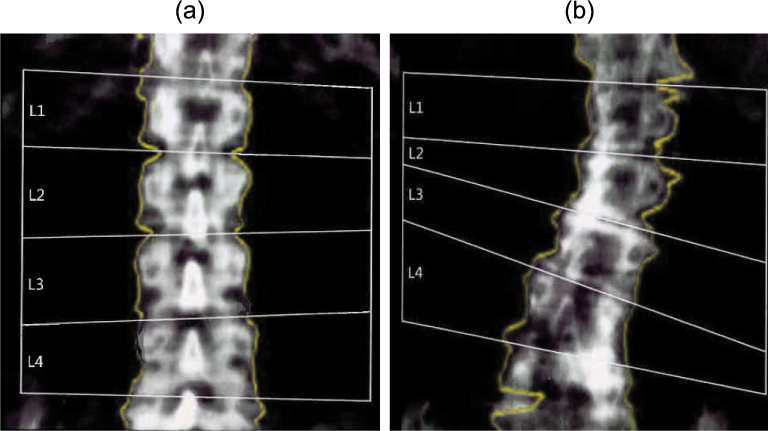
Dual-energy X-ray absorptiometry images of the spine. In DXA measurement, the bone mineral density is calculated for each semi-automatically segmented vertebra (L1-L4). (a) The subject on the left demonstrates a normal lumbar spine. (b) The subject on the right shows scoliosis and severe degenerative changes.
